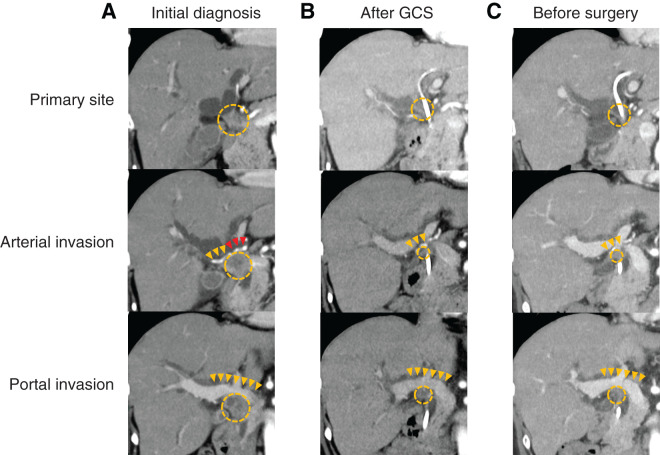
Findings of contrast-enhanced CT at initial diagnosis (A), after GCS chemotherapy (B) and before surgery (C). (A) A bile duct stricture extending from just below the hepatic duct confluence to the upper border of the pancreas was noted (dotted circle in the upper panel). A 30-mm pericholedochal lymph node metastasis was observed (dotted circle in the middle panel), along with invasion of the right hepatic artery (yellow arrowhead) and contact of left hepatic artery (red arrowhead) by the lymph node. Invasion of the main portal vein and its right branch by an enlarged pericholedochal lymph node (dotted circle in the lower panel) was observed (yellow arrowhead). (B) The stricture of the perihilar bile duct showed marked improvement (dotted circle in the upper panel). Marked shrinkage of the metastatic pericholedochal lymph node was observed (dotted circle in the middle and lower panel). The stenosis of the right hepatic artery and PV was persistent (yellow arrowhead). The contact between the metastatic lymph node and the hepatic artery disappeared. (C) The tumor remained shrunken (dotted circle in the upper panel). The size of the metastatic lymph node before surgery was persistent after GCS chemotherapy (dotted circle in the middle and lower panel). The deformation of the right hepatic artery and portal vein persisted (yellow arrowhead). GCS, gemcitabine, cisplatin, and S-1; PV, portal vein
